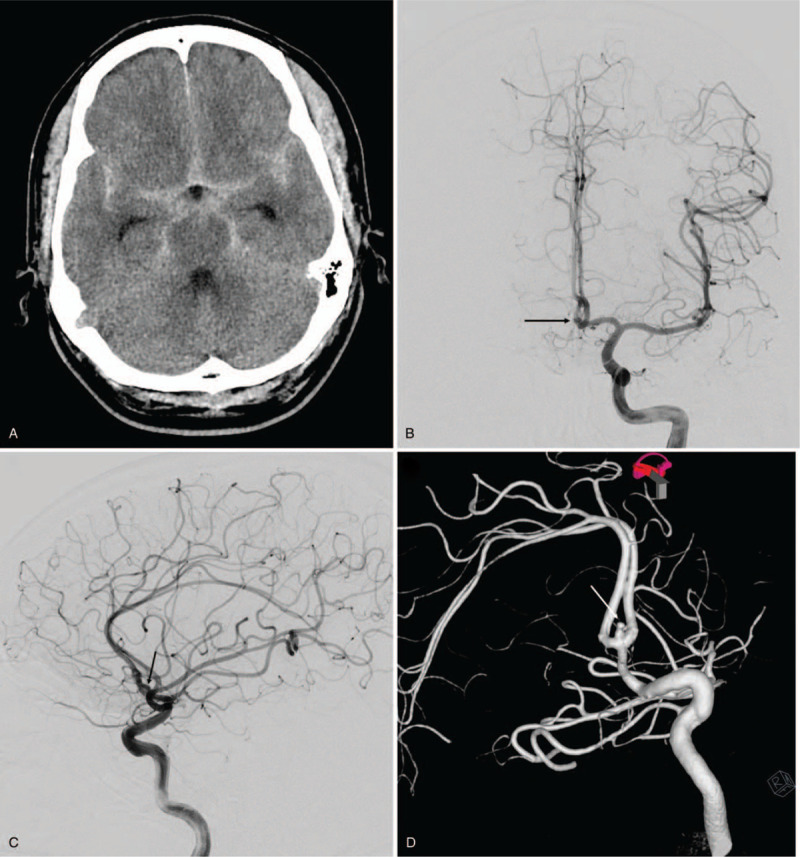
(A) Axial view of non-enhanced computed tomography showing subarachnoid hemorrhage. (B and C) Anteroposterior and lateral view of left internal carotid angiogram showing an aneurysm originating at the anterior communicating artery (black arrow). (D) Volumetric 3-dimensional reconstruction of digital subtraction angiography showing a snowman-like shaped aneurysm on working projection (white arrow).
